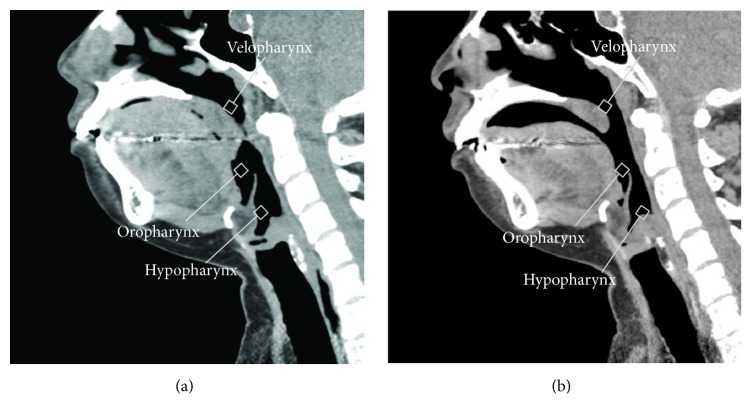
Mid-sagittal CT sections of the pharynx in patient 1 (a) before UPPP and (b) after UPPP.
